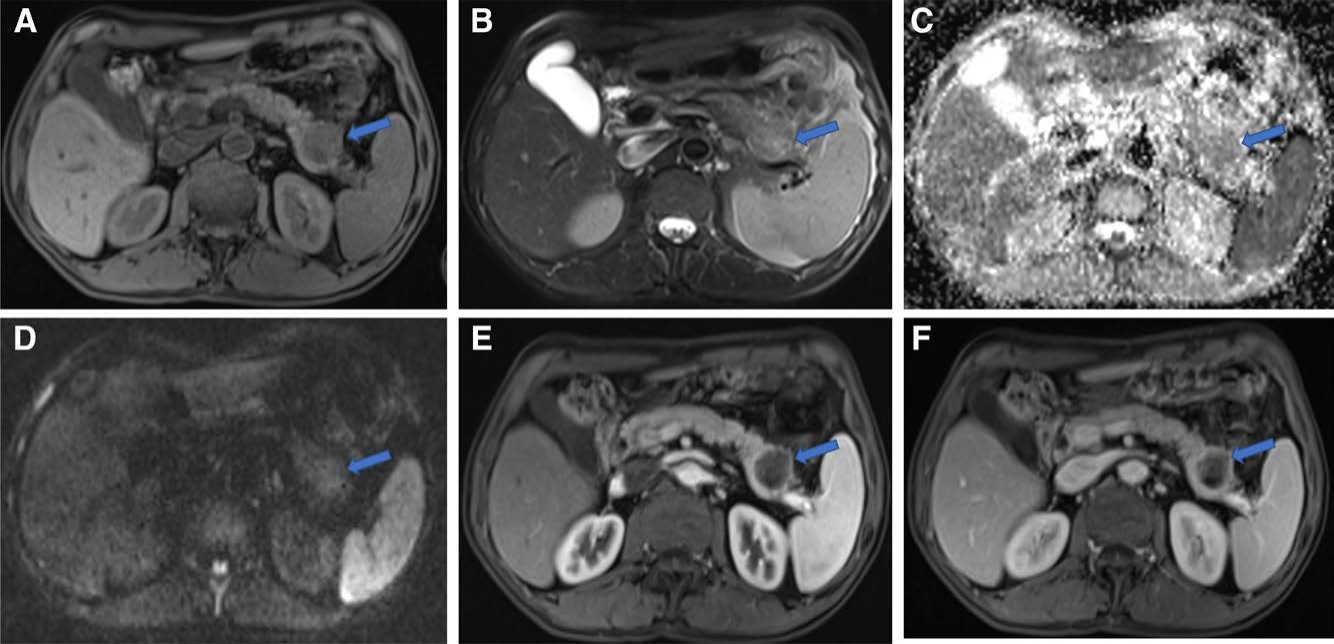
October 22, 2021 upper abdominal contrast-MRI, demonstrating the lesion in the tail of the pancreas, with 3.1 cm × 2.9 cm mass, slightly low signal on T1WI (A), mixed slightly high signal on T2WI (B), and had clear boundary, mild limited diffusion, the mean ADC value is approximately 1154 × 10⁻³ mm²/s (C, D), and rim enhancement on enhanced images (E, F). ADC = apparent diffusion coefficient, MRI = magnetic resonance imaging.
